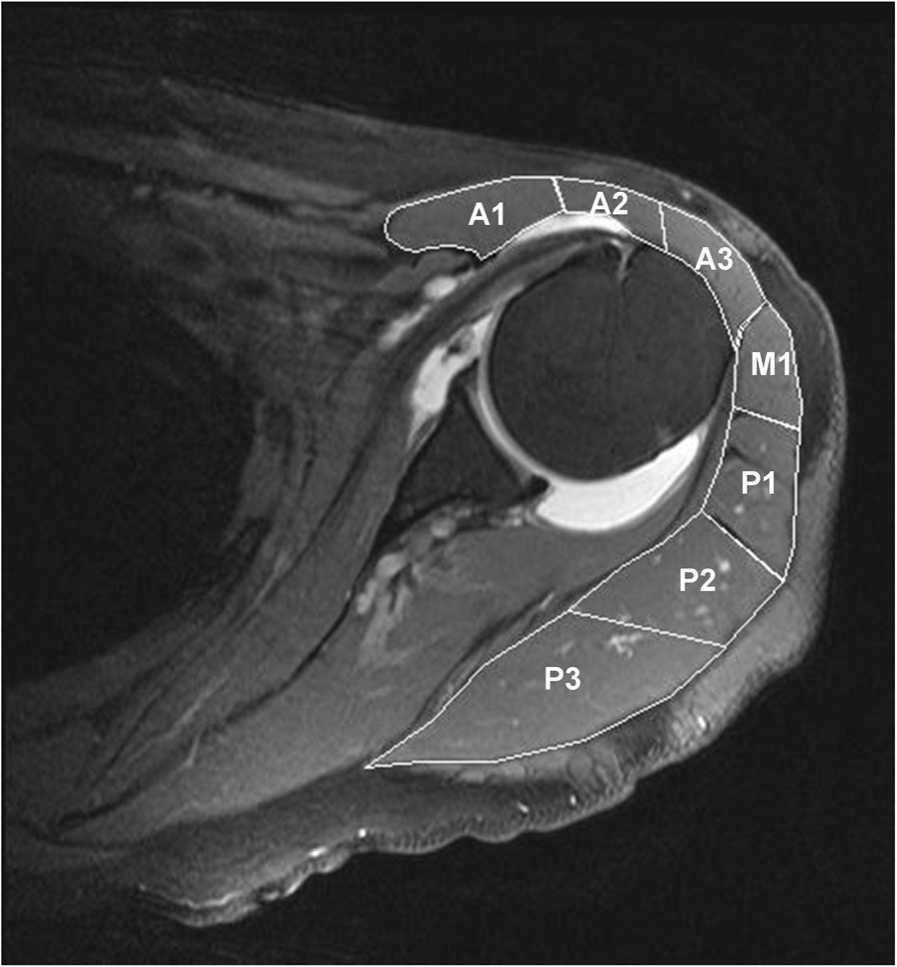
Area of anatomic deltoid muscle segments. On T2 weighted transverse MR images the area of seven anatomic defined segments of the deltoid muscle were measured exactly at the mid-glenoid level. These segments were identified following the visible tendons from their origin-points of the clavicle (A1), the anterior surface of the acromion (A2), the lateral aspect of the acromion (A3, M1 and P1) and the scapular spine (P2 and P3) respectively.
